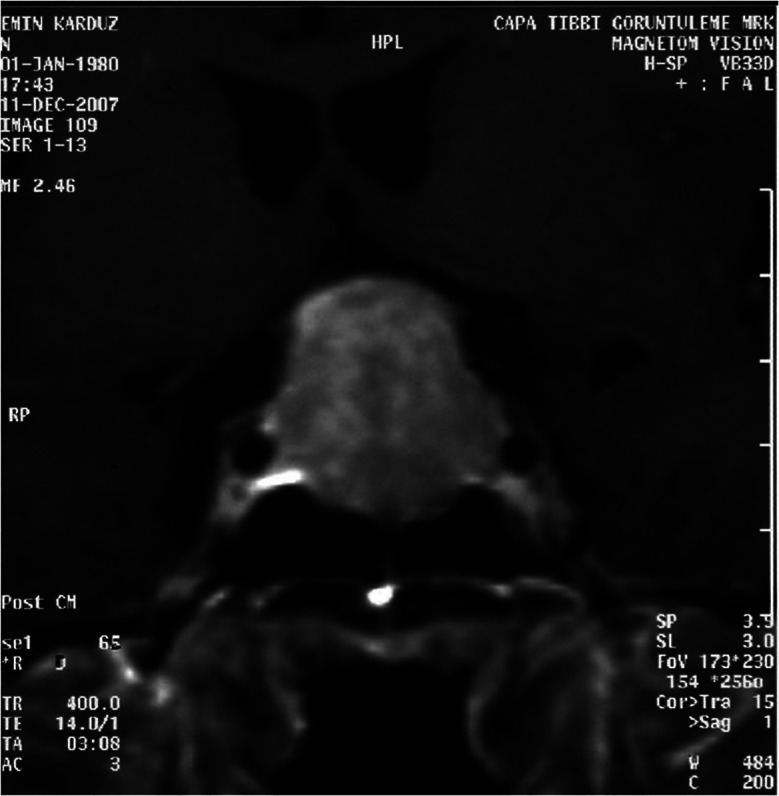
Preoperative sella MRI of the patient indicating a 3 × 2.8 × 2.2 cm intrasellar mass extending superiorly.
